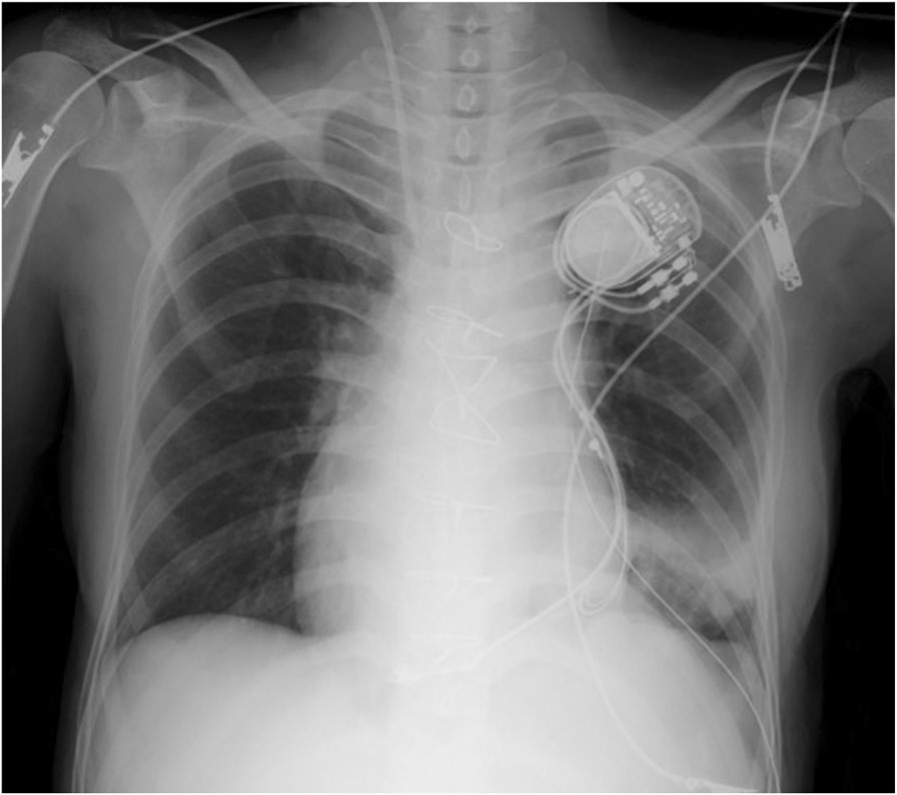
The postoperative chest X-ray after the extubation showing the successful re-expansion of the left lung
